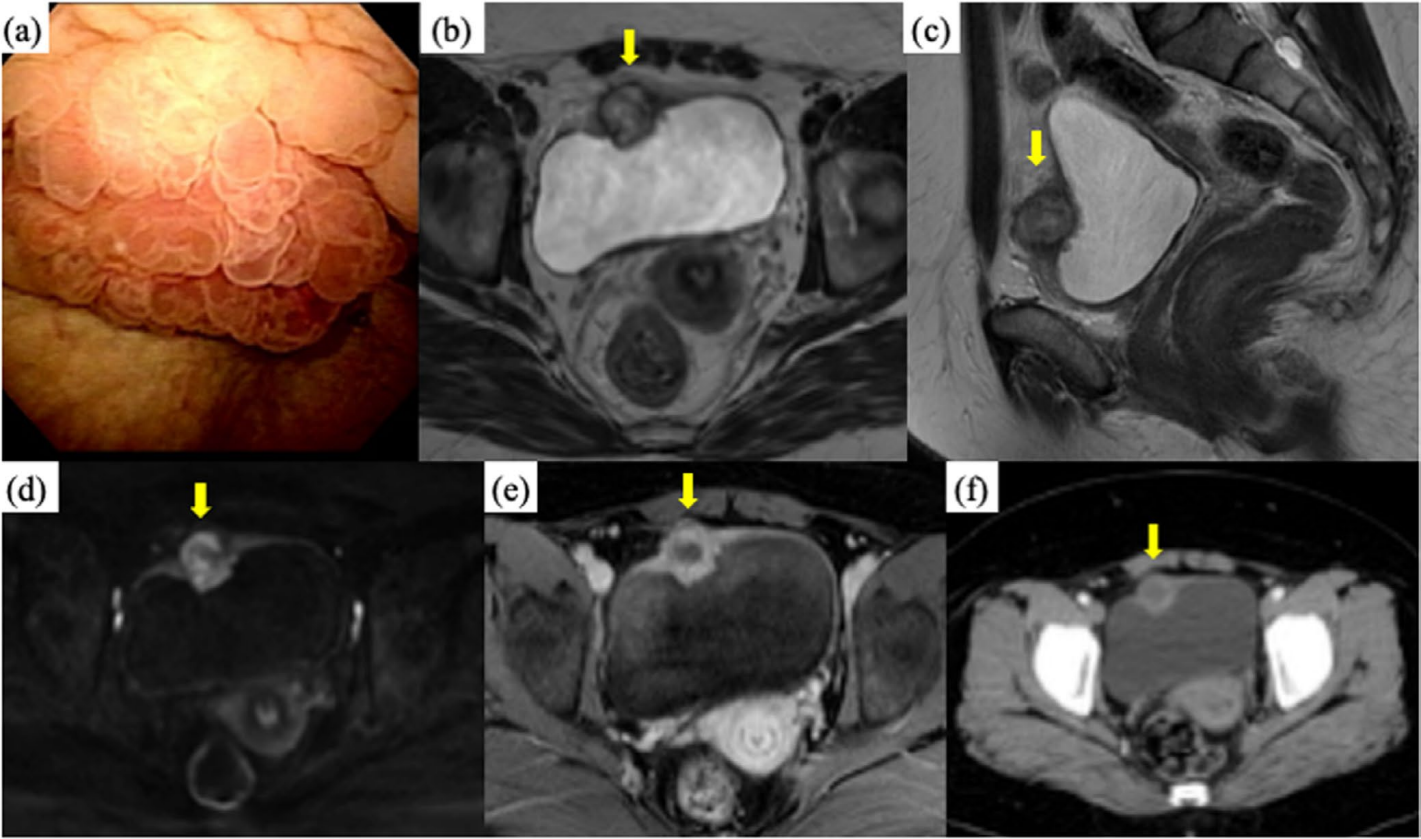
Cystoscopy showed an edematous tumor at the anterior wall of the bladder (a). Bladder mass with iso‐signal on T2‐weighted magnetic resonance imaging (b, c). Diffusion‐weighted imaging (DWI) and contrast‐enhanced MRI showed ring‐like or peripheral enhancement (d, e). Contrast‐enhanced CT exhibited similar characteristics (f).
